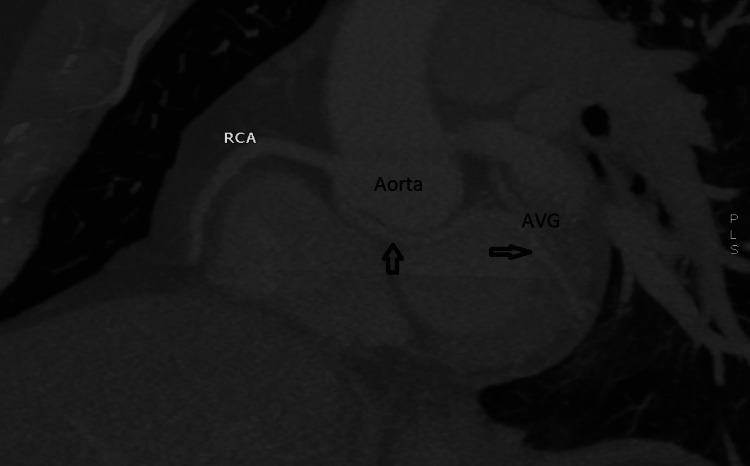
Cardiac CT with 3D reconstruction. The two black arrows indicate a retroaortic artery arising from RCS near the right coronary artery directed toward the atrioventricular groove. RCS: Right coronary sinus; RCA: Right coronary artery (925*489 DPI 300)
